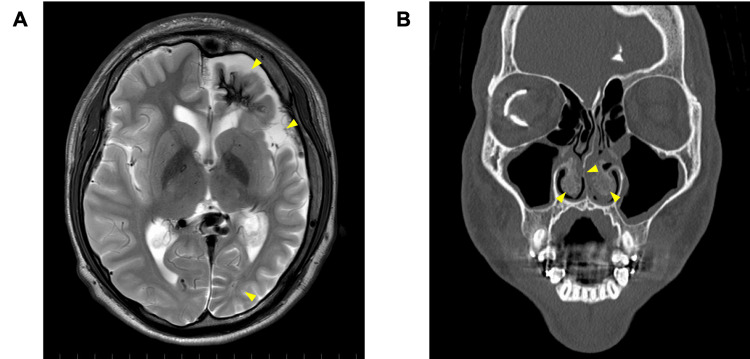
Presurgical evaluation. (A) Fat-suppressed T2 weighted magnetic resonance image. The arrowhead shows the atrophy of the left cerebral hemisphere which is typical symptom of SWS. (B) Computed tomography image. The arrowhead shows that the inferior turbinate was thickened and the nasal septum had deviated. This makes nasal intubation difficult.
